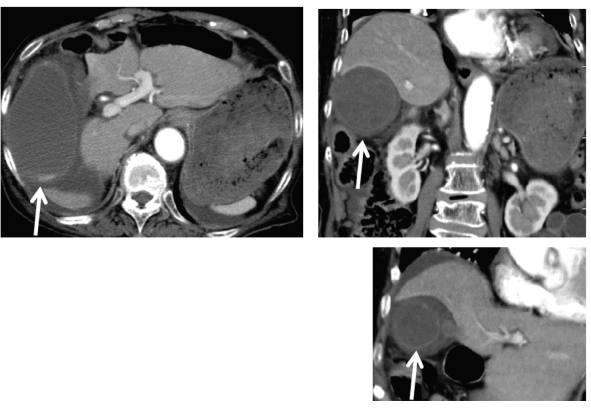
Abdominal enhanced computed tomography showed enlarged gallbladder and thickness of the gallbladder wall, and ascites around gallbladder.
